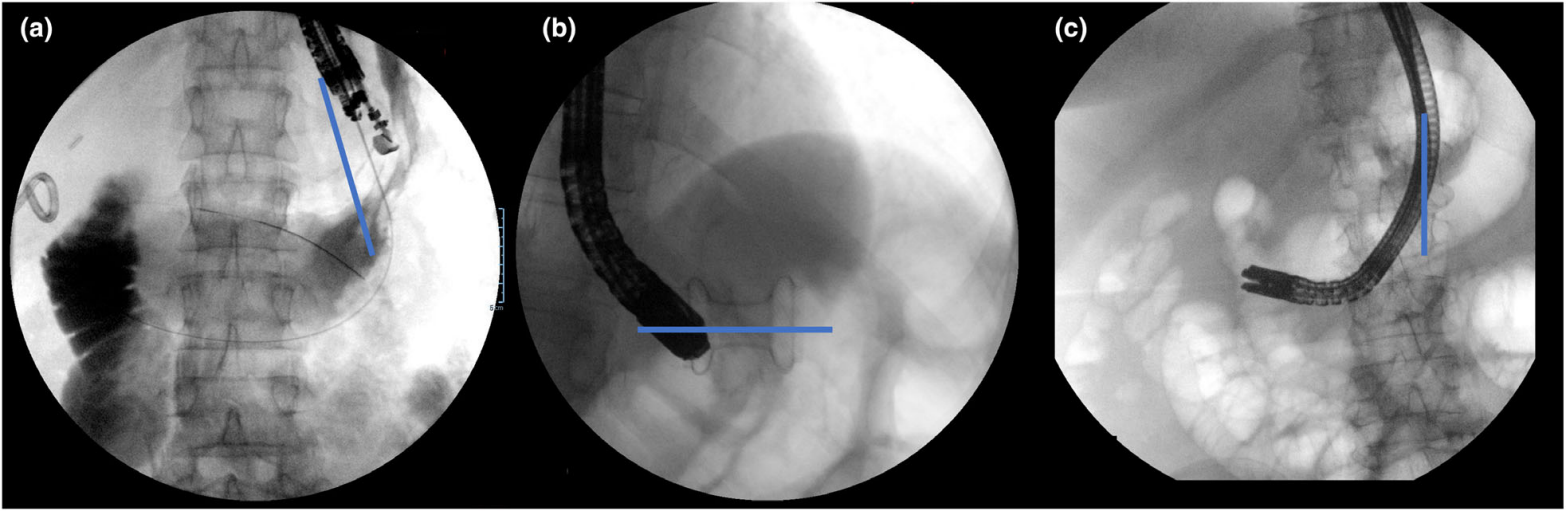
Three endoscopic ultrasound‐guided anastomosis. (a) Gastro‐gastric anastomosis in the middle‐excluded stomach with an oblique axis. (b) Tentative of passing through a gastro‐gastric anastomosis in the proximal stomach. The horizontal axis of the stent located in the subcardial region leads to a difficult manipulation of the duodenoscope. (c) A narrow space in the excluded antrum of a patient who underwent a jejuno‐gastric endoscopic anastomosis with a vertical axis leads to a difficult positioning of the scope during the intervention.
